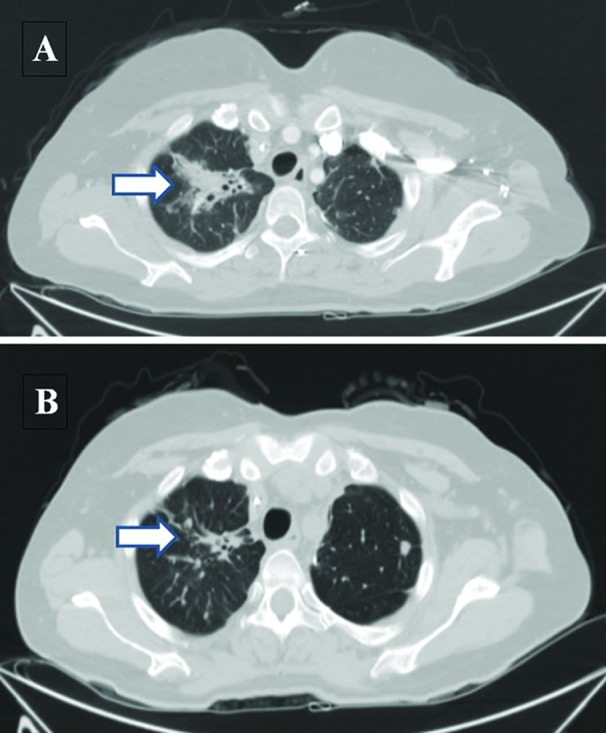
Representative CT scan images of upper lung metastasis at baseline (A) and after 3 months of trastuzumab and chemotherapy (B) Arrow indicates significantly regressed tumor burden. (Note: respiratory variability accounts for slight anatomic differences in pre/post imaging).
